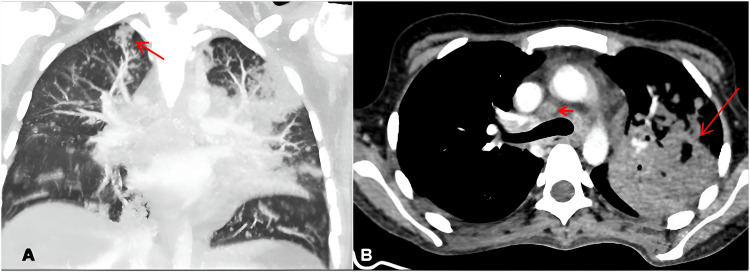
Patient's chest CT scan - Case 1 Chest CT images exhibiting diffuse centrilobular micronodules in the coronal view (Figure 1A), showing a tree-in-bud (TIB) pattern in the upper right lobe (red arrow); axial image (Figure 1B) showing mediastinal and hilar lymphadenopathy, measuring up to 18 mm in its smaller axis (arrowhead), as well as consolidation surrounded by adjacent restrictive pulmonary atelectasis in the upper left lobe (red arrow).
